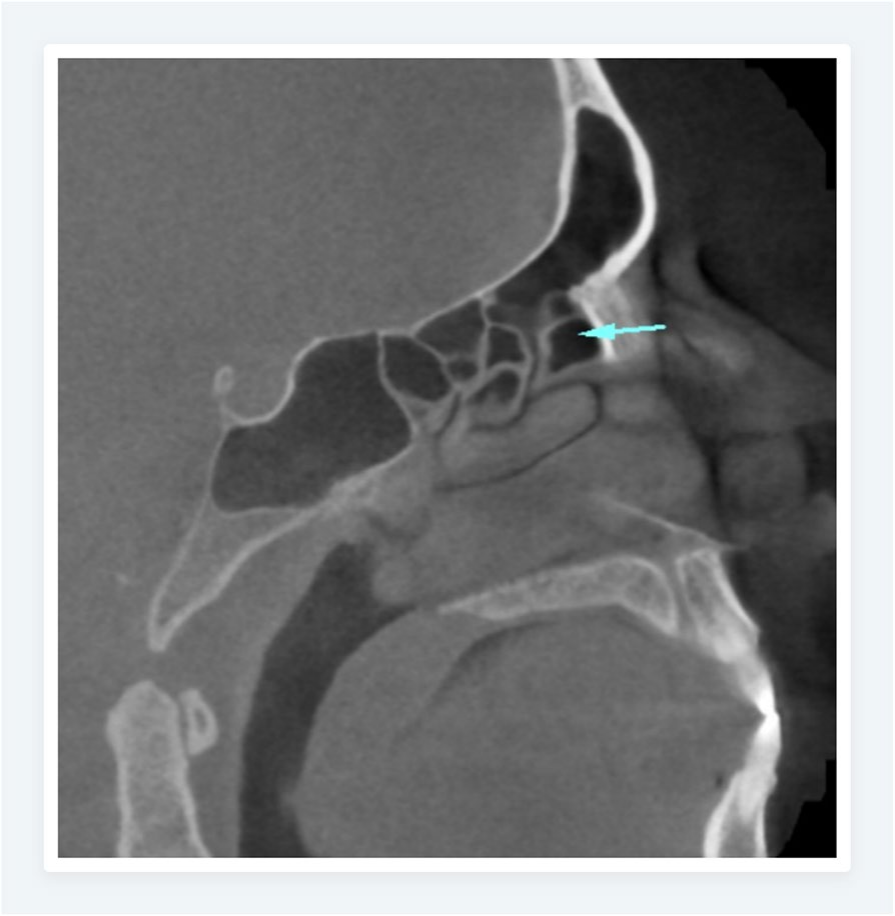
Sagittal CBCT plane showing agger nasi cell (arrow) and its relation with the frontal sinus ostium
